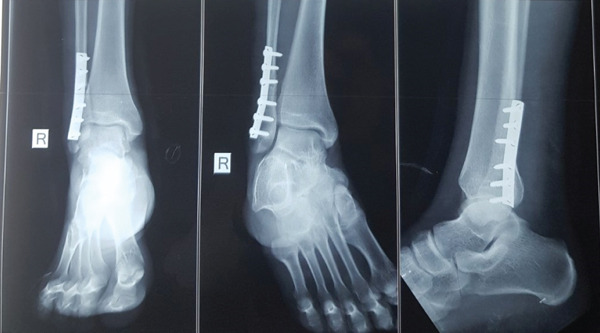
Anteroposterior, mortise, and lateral plain radiographs showing fixation with a one‐third‐tubular plate with six screws.
